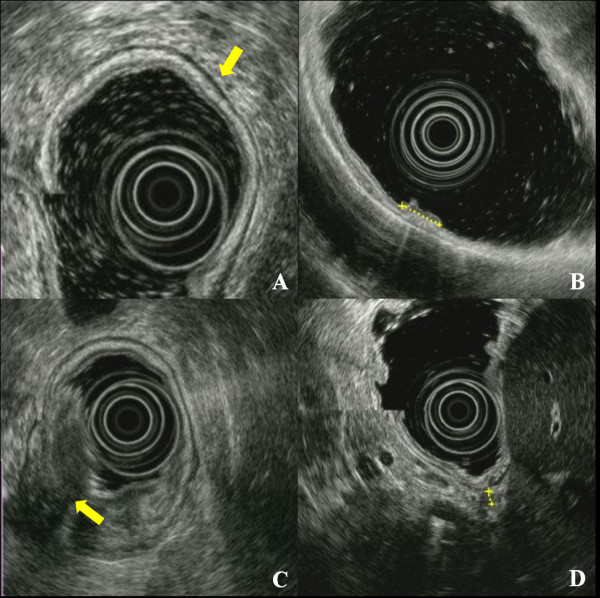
Endoscopic ultrasonography (EUS) (A) EUS showing normal layers of gastric wall, with the five-layered echo pattern being clearly visible (arrow). (B) EUS of early gastric cancer (T1a), showing a focal thickening area confined to layers 1 and 2(dotted line). (C) EUS image of advanced gastric cancer (T3); the tumor involves all layers and extends beyond the the outermost layer of the gastric wall (arrow). (D) EUS showing a metastatic lymph node (dotted line).
